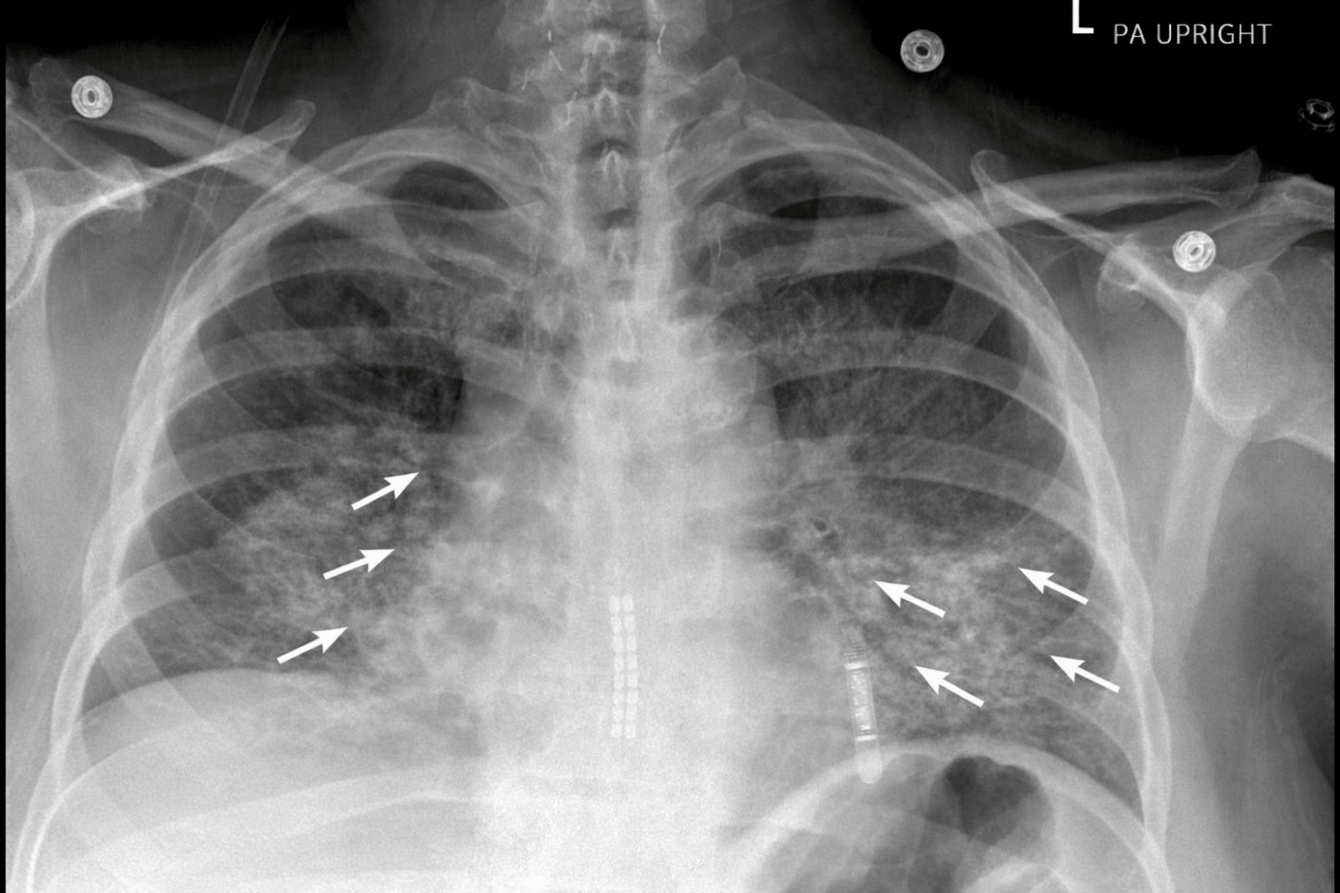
CXR on hospital day 4: new bilateral infiltrates with vascular congestion. CXR: chest radiograph
